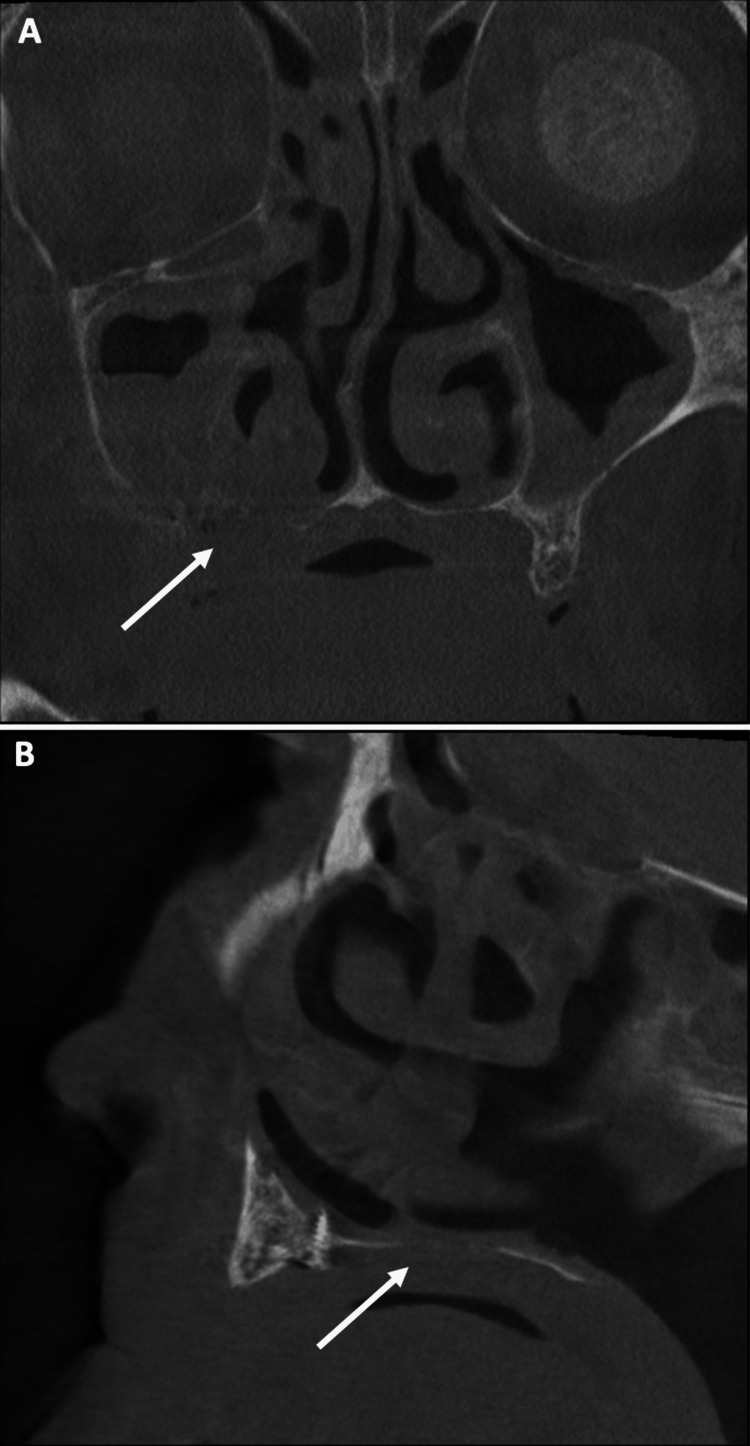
Situation at four months in (A) frontal view and (B) sagittal view of CT scan.
